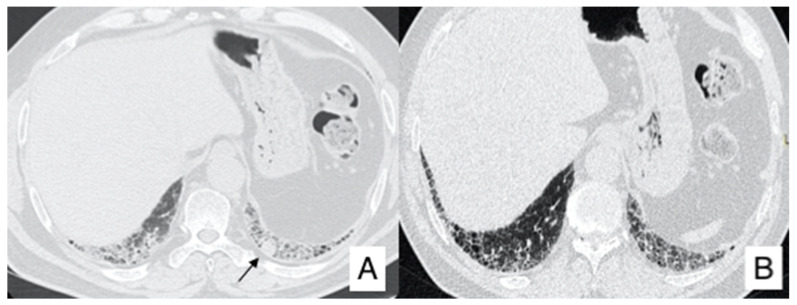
One year later, follow-up HRCT of the same patient in Figure 6, identified a nodule in the left lower lobe (black arrow in (A), that was not shown in the first examination (B).
